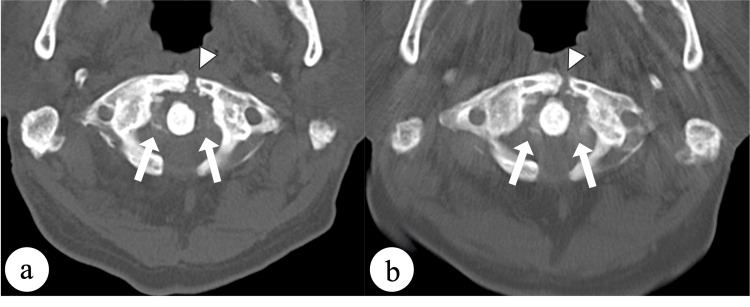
Findings on follow-up axial computed tomography scans. (a) Image obtained at three years postoperatively showing filling of the fracture gap (arrowhead) and calcification of the transverse ligament (arrows). (b) Image obtained five years postoperatively showing persistence of nonunion (arrowhead) and progressive calcification of the transverse ligament (arrows).
